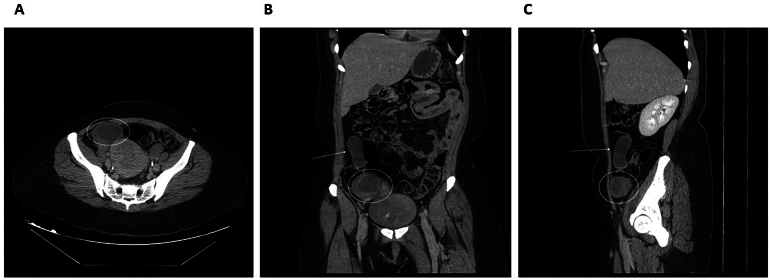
CT scan showing appendicular mass and elongated cystic lesion. A. Axial view, B. Coronal view, C. Sagittal view.
